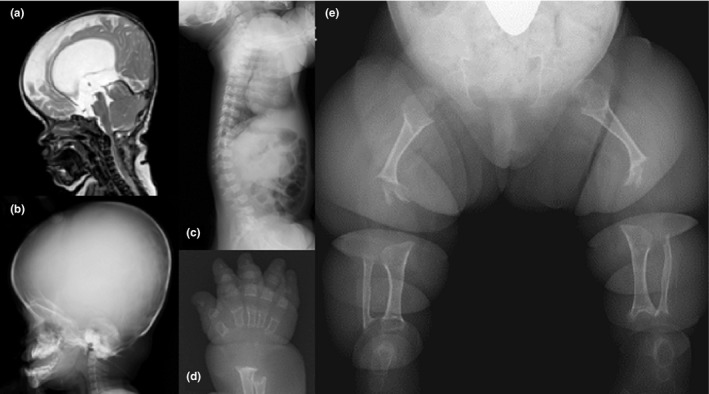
Image findings during infancy. Magnetic resonance imaging of the head and cervical spine at the age of 3 months indicated hydrocephalus and foramen magnum stenosis (a). Lateral radiograph of the skull at the age of 1 year showed a large skull with relatively small cranial base, hypoplastic maxilla, and protruded mandible (b). Lateral radiograph of the spine at the age of 1 year demonstrated mild platyspondyly with tongue‐like protrusion of the lumbar spine and extremely shortened ribs with metaphyseal flaring (c). Anteroposterior radiograph of the right hand at the age of 1 year showed generalized brachydactyly with marked metaphyseal irregularities (d). Anteroposterior radiograph of the lower limbs at the age of 2.7 years demonstrated extremely shortened and thin long tubular bones with deficient ossifications at the metaphysis and epiphysis (e)
